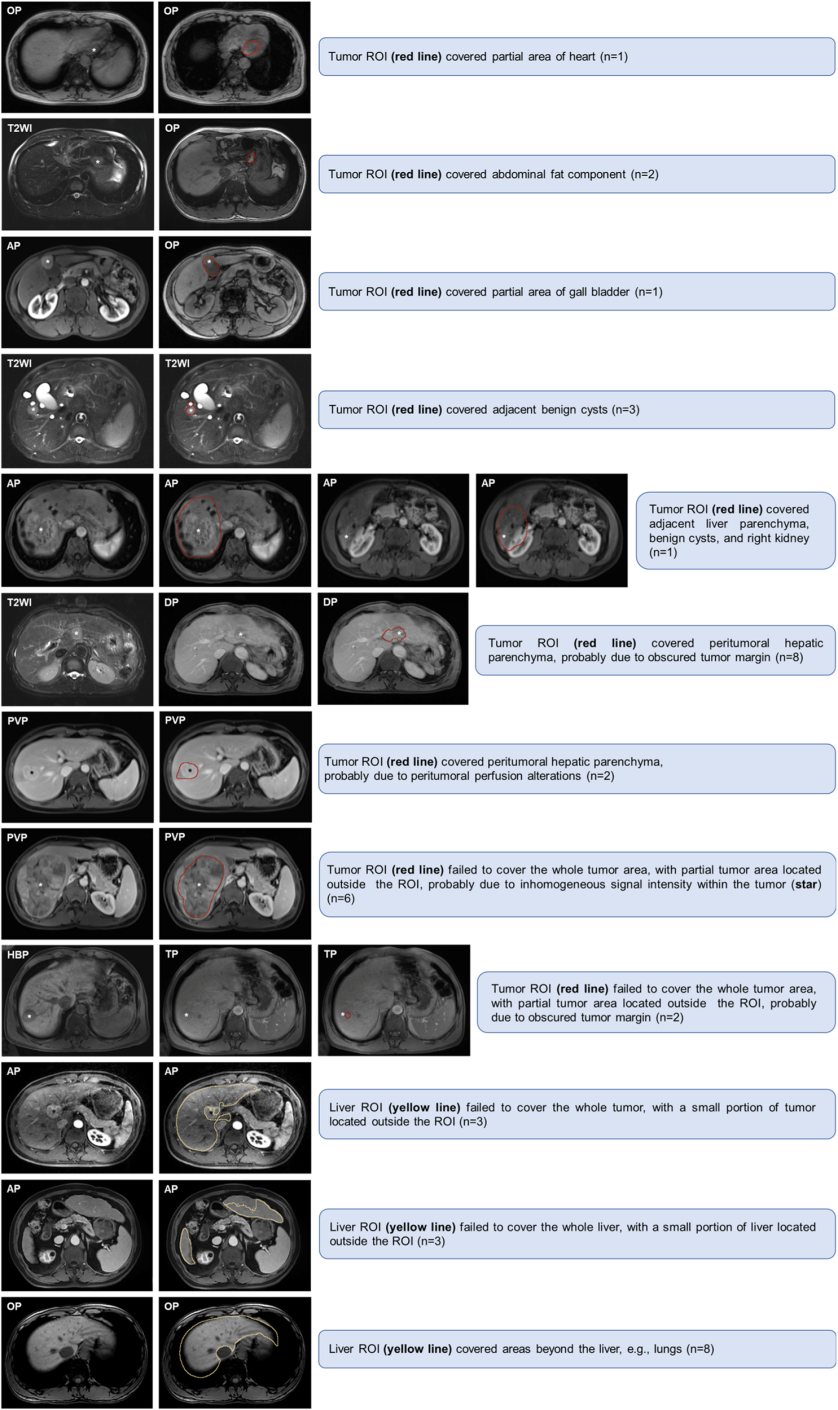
Examples of inaccurate image segmentations. AP, arterial phase; DP, delayed phase; OP, opposed phase; PVP, portal venous phase; ROI, region of interest; TP, transitional phase; T2WI, T2-weighted imaging
